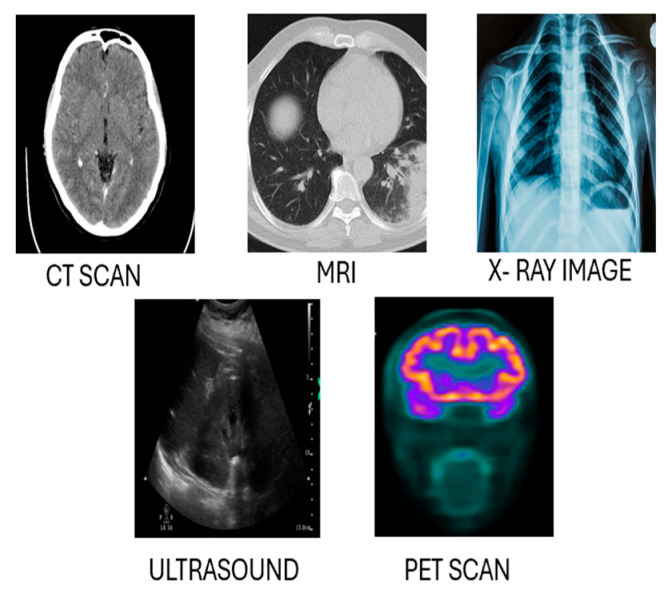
A comparison of different radiology techniques: X-ray imaging, PET (Positron Emission Tomography), CT (Computed Tomography), Ultrasound (US), and MRI (Magnetic Resonance Imaging).
